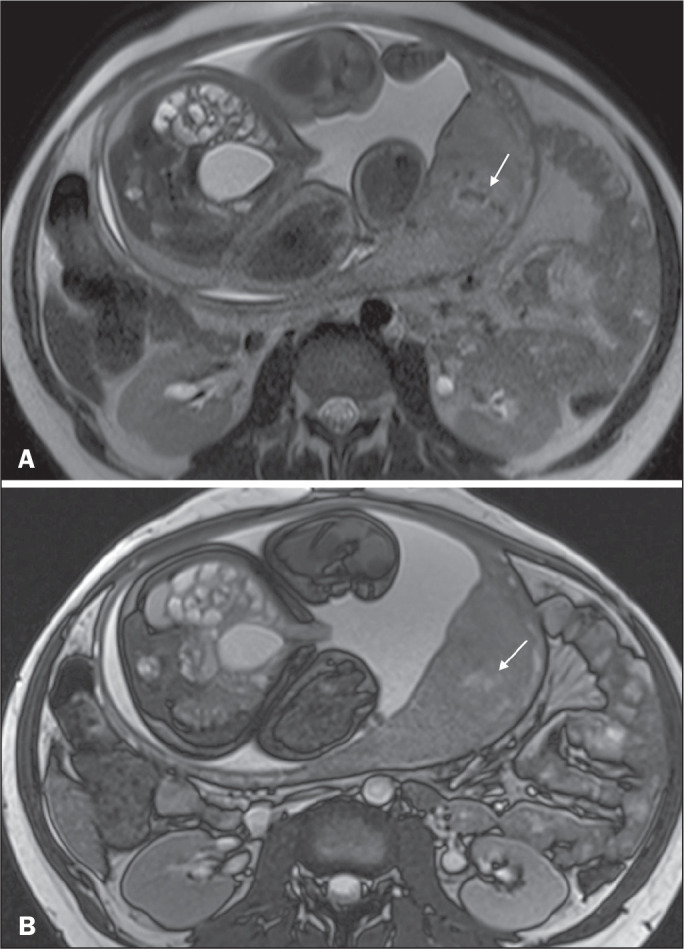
A 33-year-old patient. Axial T2-weighted HASTE and TrueFISP sequences (A
